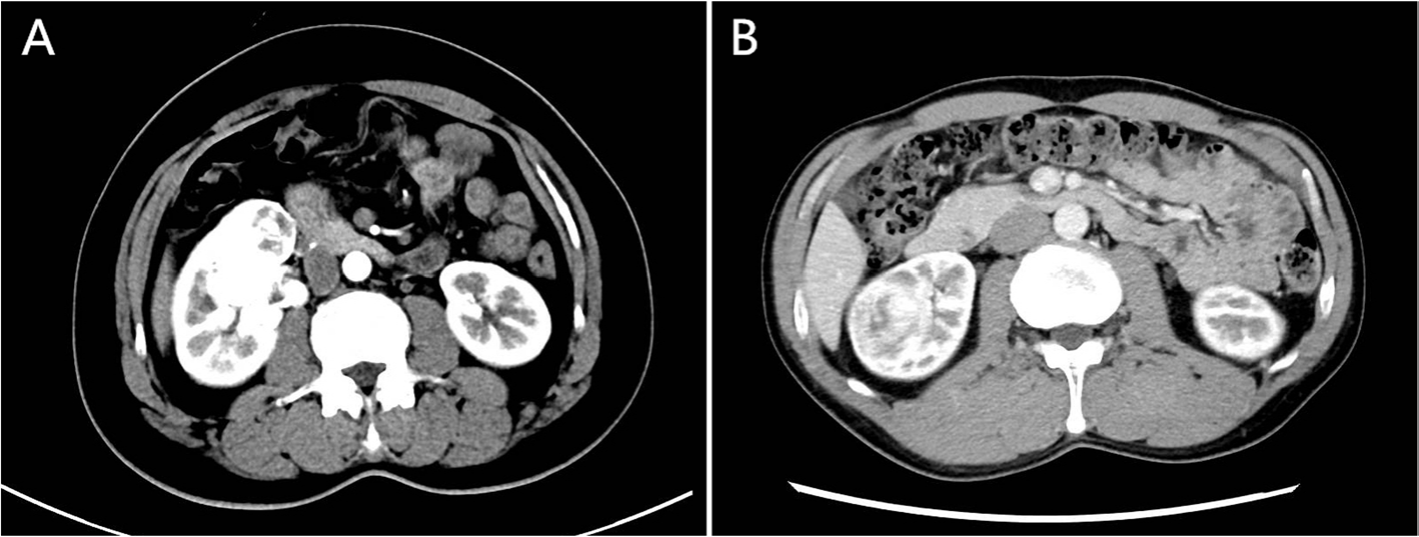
CT findings of complex renal cell carcinoma. A Intermediate complexity renal cell carcinoma (PADUA score: 9). B High complexity renal cell carcinoma (PADUA score: 11)
